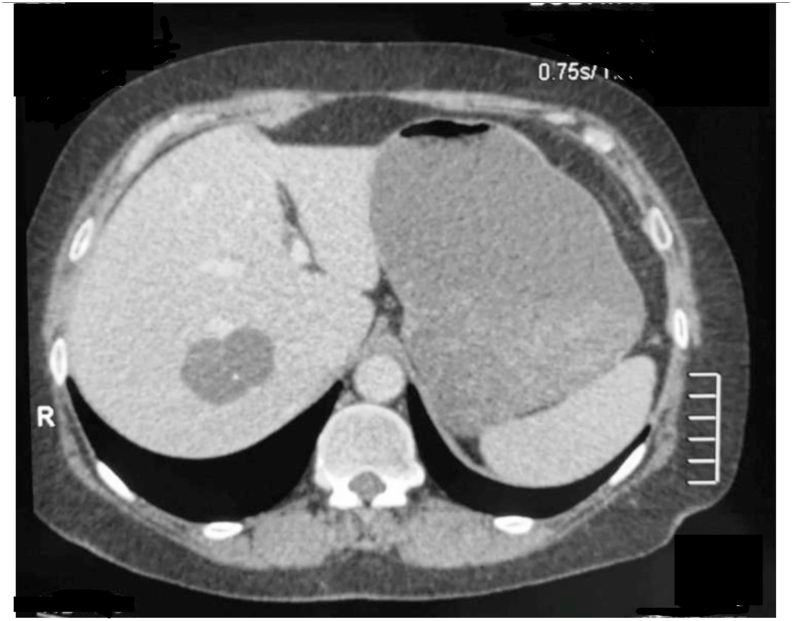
CT scan of the preoperative period. There is the dumbbell-shaped cyst of 4.3*3.1*3.7 cm with heterogeneous structure in the hepatic segment VII.
